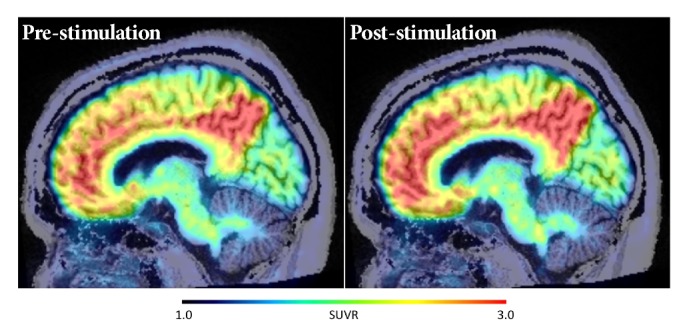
Average ^11^C-PiB SUVR images of the six subjects in the pre- and poststimulation conditions. No significant differences were seen anywhere in cortical regions.
